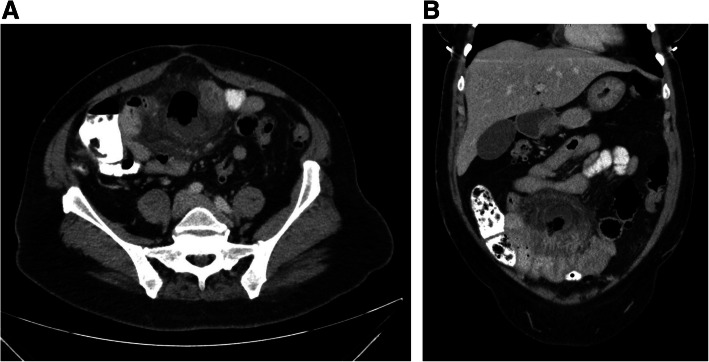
CT of the abdomen with oral and IV contrast. a Axial image of the mid-jejunal diverticulum with perforation. b Coronal image demonstrating associated edema of the jejunal loop and adjacent mesentery
